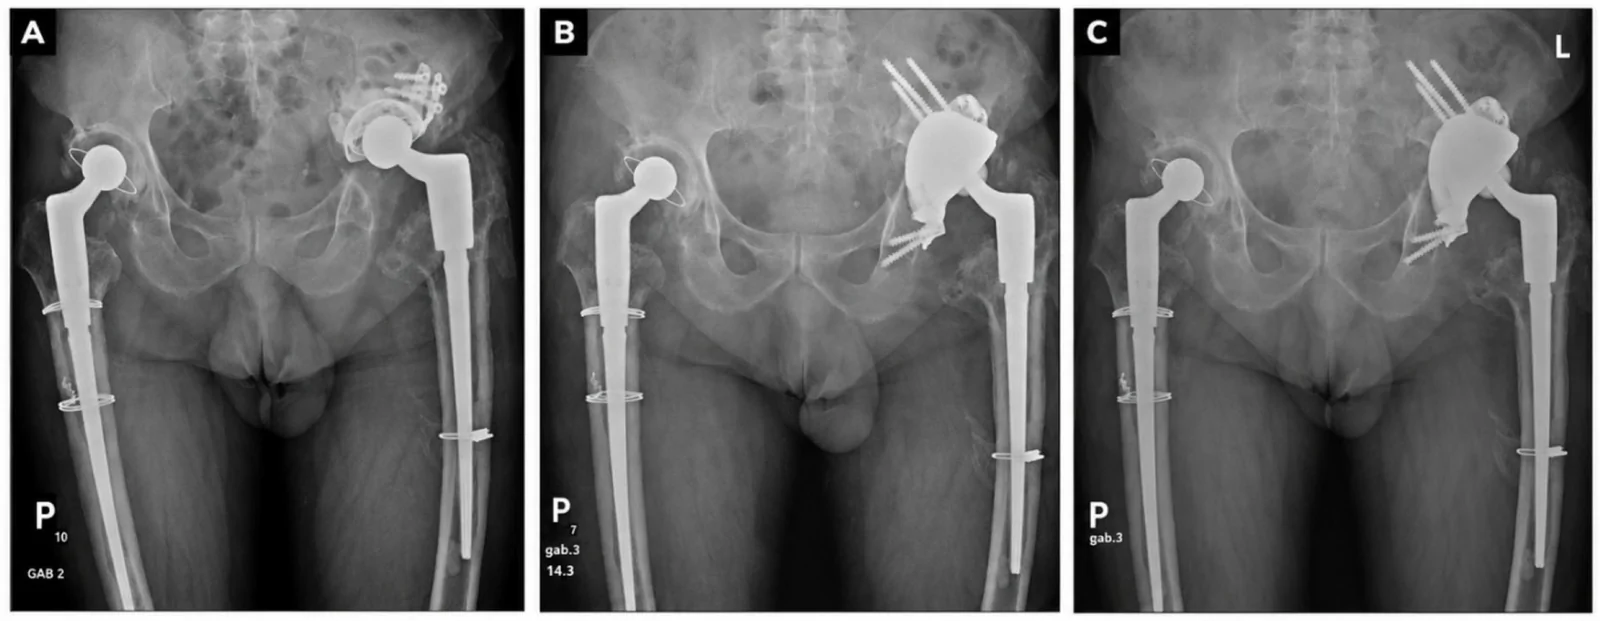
Representative case of a Paprosky type 3B acetabular defect treated with a custom-made 3D-printed implant. (A) Preoperative radiograph demonstrating superior migration of acetabular component with extensive bone loss. (B) First day after surgery radiograph—3D custom-made reconstruction and (C) one year after surgery demonstrating stable implant position and no sign of implant subsidence or loosening. “P” denotes the right side (“prawa” in Polish), and “L” denotes the left side (“lewa” in Polish).
